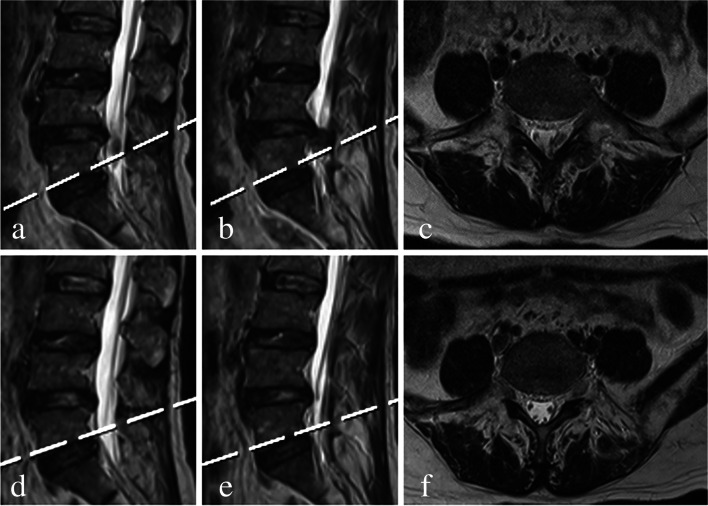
a, b Preoperative sagittal and (c) axial T2 MRI showing a very highly down-migrated disc from L4 to L5. d, e Postoperative sagittal and (f) axial T2 MRI showing successful surgical removal of the downward migrated fragments
